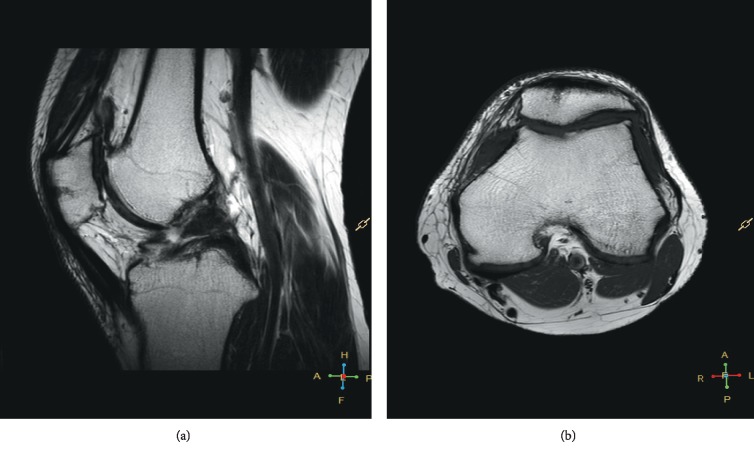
(a) Follow-up MRI T2 sagittal—osteophytes in the patellofemoral joint in one of the three patients with degenerative changes. (b) Follow-up MRI T1 axial in the same patient.
